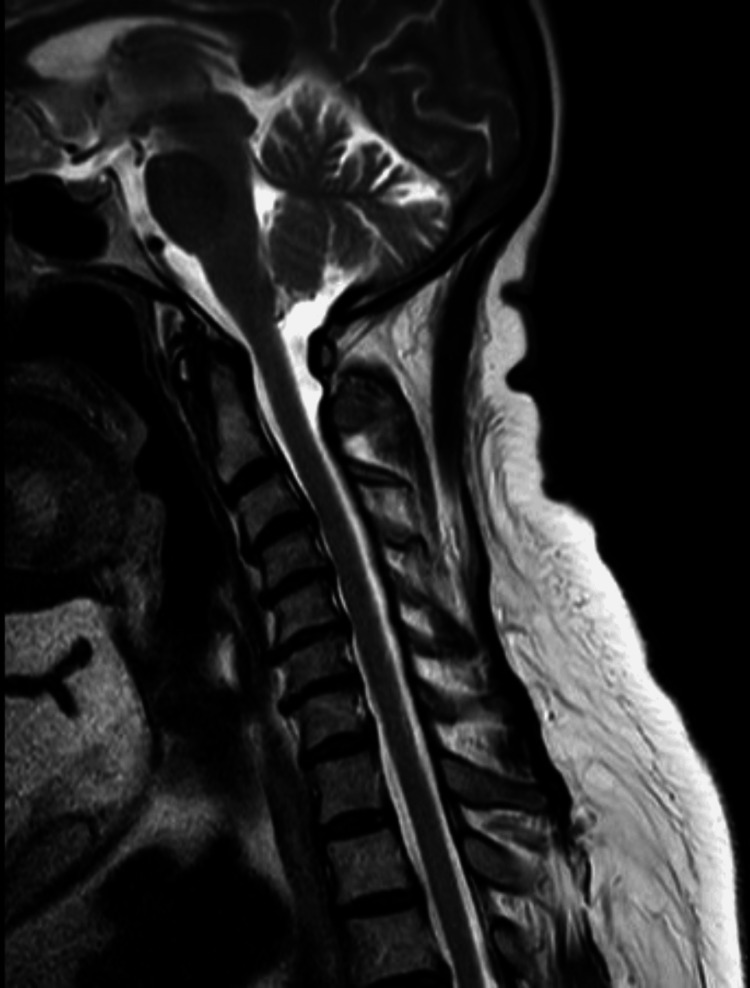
MRI of the cervical spine MRI of the C-spine was done to rule out cervical myelitis as the patient was complaining of lower neck pain.
